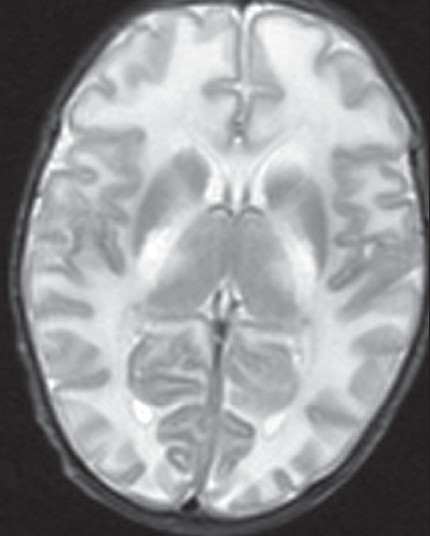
MRI (T2W) in a 14 days- old baby with classic maple syrup urine disease. Note the diffuse symmetrical white matter hyper intensity of white matter. In addition, note involvement of globus pallidi and thalami
